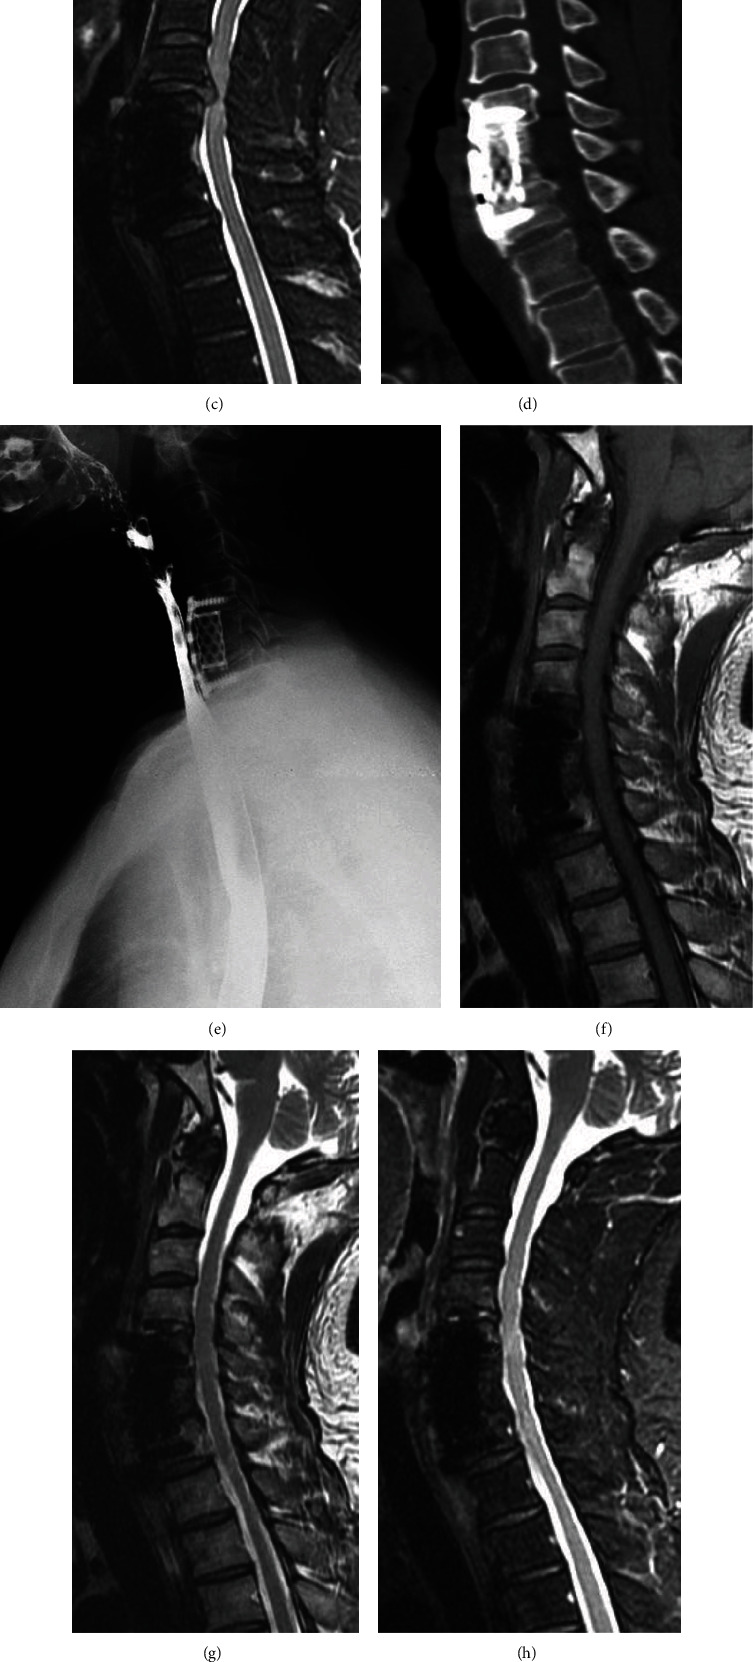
54-year-old male patient who at 3 years after anterior cervical corpectomy and fusion (ACCF) surgery complained of weakness in both lower extremities and numbness of both hands for two months. Preoperative T1- (a), T2- (b), and fat suppression- (c) weighted MR images and CT scan (d) show a huge disc herniation adjacent to previous fused C5-7 segments. Preoperative esophageal barium meal examination (e) shows a slight anterior protrusion of the posterior wall of the esophagus caused by compression and adhesion of the anterior cervical plate. Anterior revision surgery using ROI-C device was successfully performed and postoperative T1- (f), T2- (g), and fat suppression- (h) weighted images show complete decompression of the C4-5 segment. Postoperative anteroposterior (i) and lateral (j) radiographs show favorable ROI-C device location.
